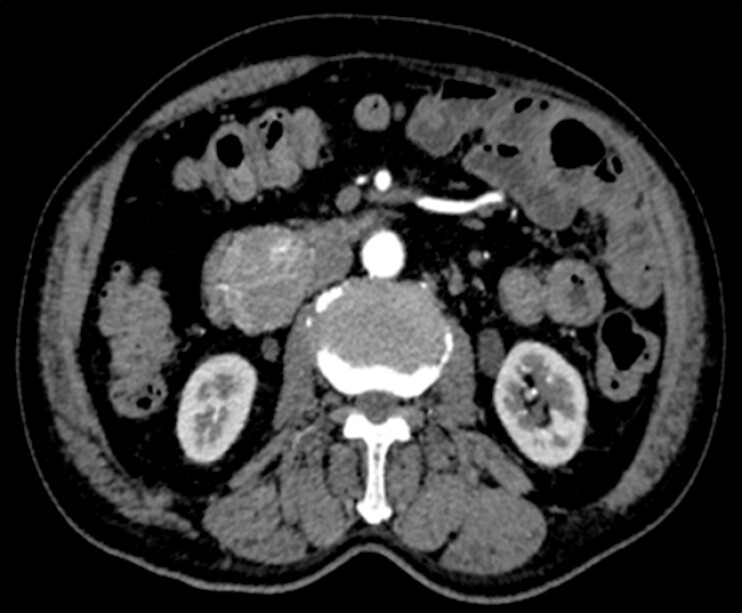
Computed tomography showing a hypervascular lesion in the descending portion of duodenum.
